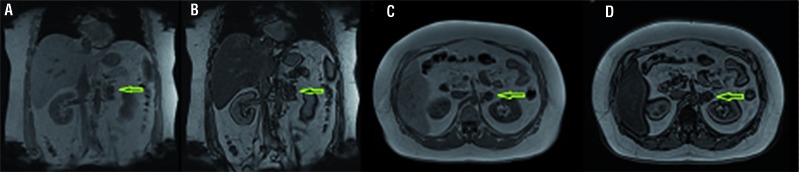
Magnetic resonance images (ECO Gradient sequences GRE) T1W1 inside and outside of phase (A and B) and in T2 with and without fat suppression (C and D); they show sparse areas of signal loss corresponding to lipomatous foci.
